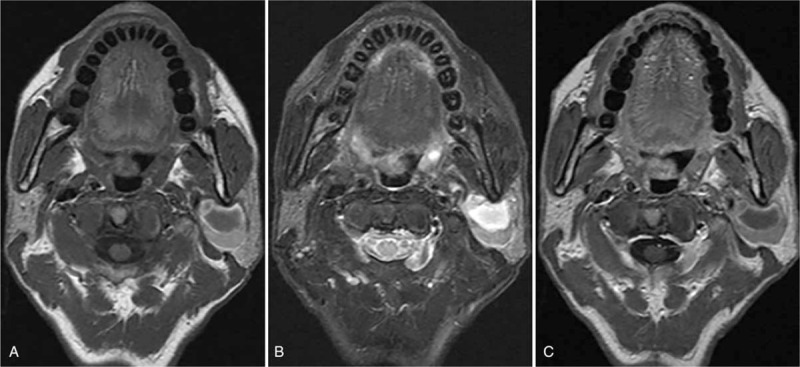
A 62-yr-old man with myopericytoma in the left parotid gland. (A and B) T1-weighted images (A) and T2-weighted images (B) revealing a well defined, cyst-solid mixed mass with slightly high signal intensity in the solid portion; (C) Axial enhanced scan revealing no enhancement of the lesion. The areas of necrosis and cystic degeneration were non-enhanced.
